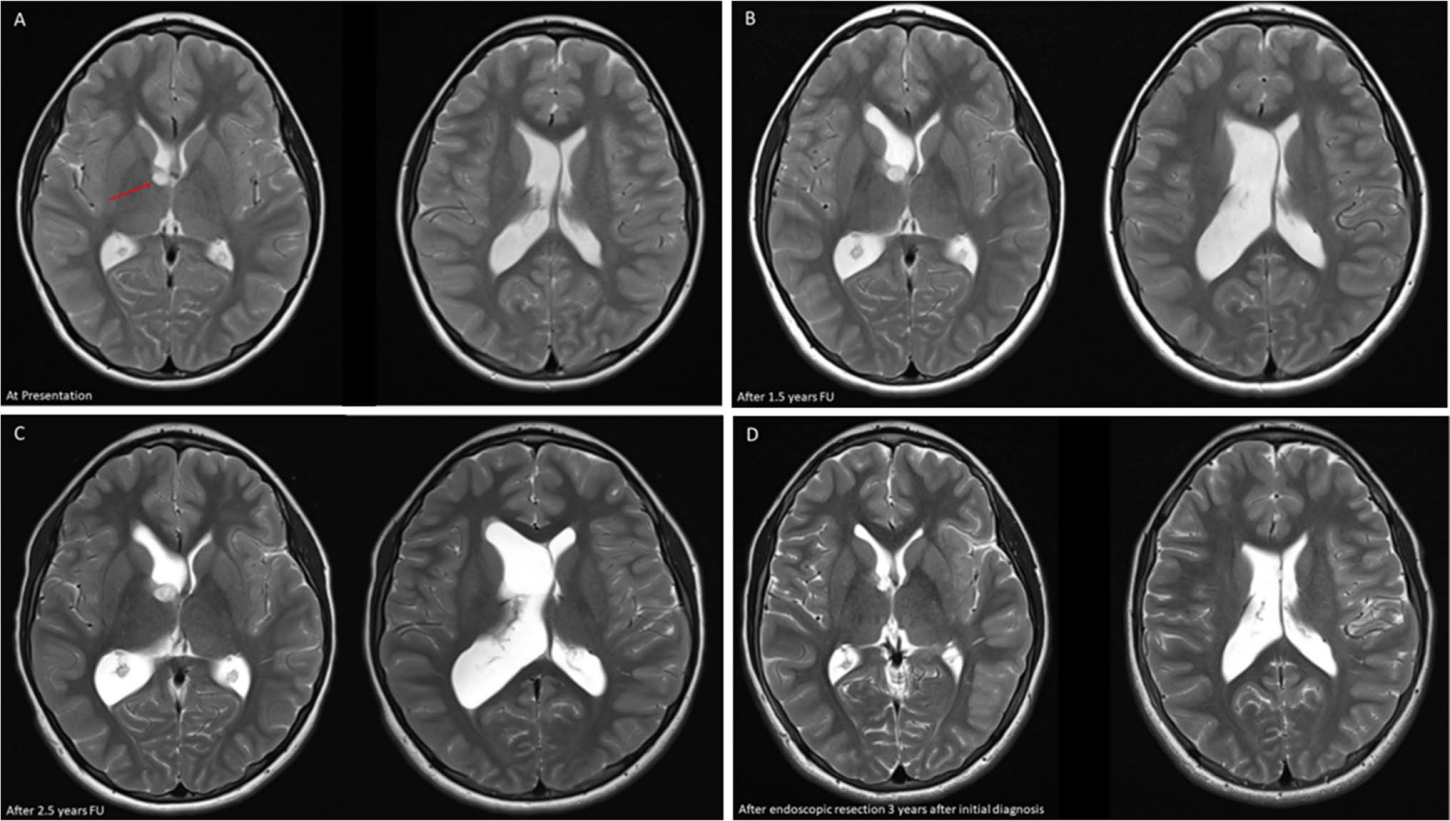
Example of a supratentorial incidental brain tumor finding eventually managed surgically A 11 year old boy presenting with growth retardation. On MRI paraventricular incidental finding within the basal ganglia. Initially a conservative management with wait scan follow up was initiated B and C Due to minimal growth of the lesion and clear progression of the ventricular size, an endoscopic transventricular removal of the lesion was undertaken, showing a low grade glial/glioneuronal tumor D 3 years postresection no recurrence or residual tumor is seen
